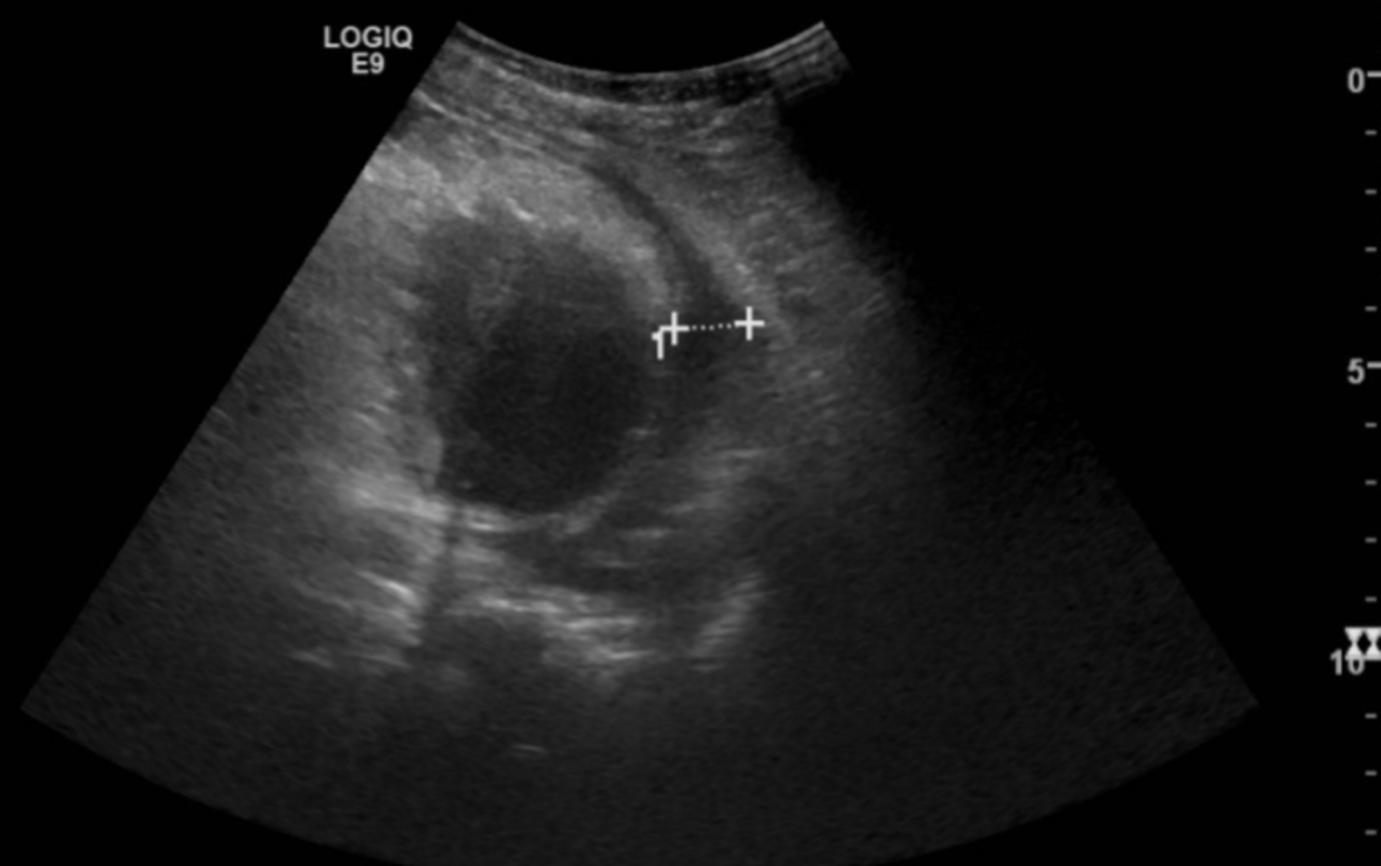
Chest ultrasound showing moderate to large pleural effusion.
